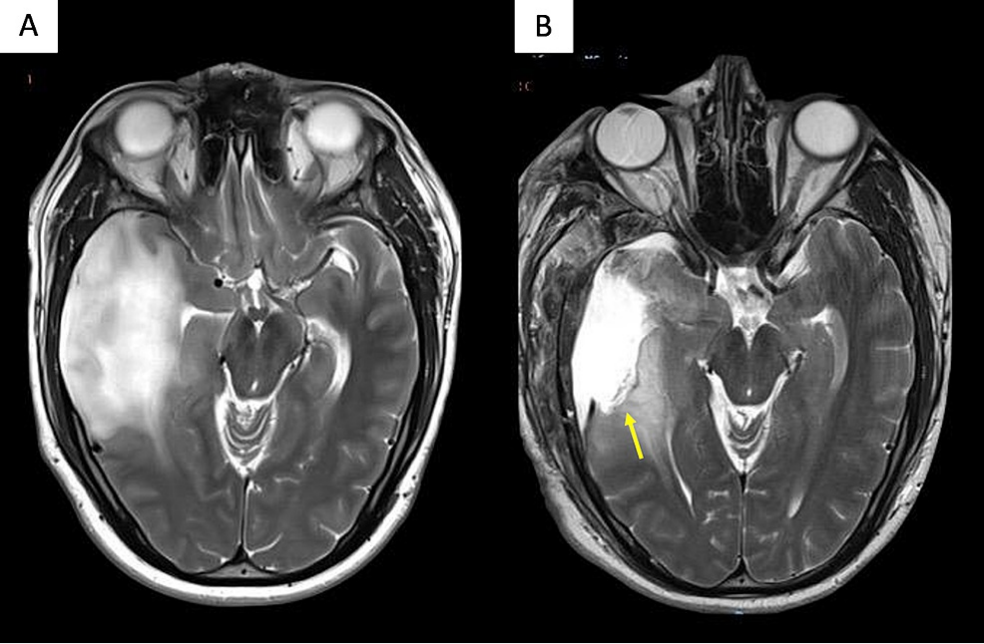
Preoperative and postoperative T2 sequence MRI. (A) Preoperative and (B) Postoperative T2 sequence MRI showing residual tumor on the most caudal and medial portion of the lesion (yellow arrow), which exactly corresponds with the optic radiations pathway and marks the limit of our cortical mapping.
